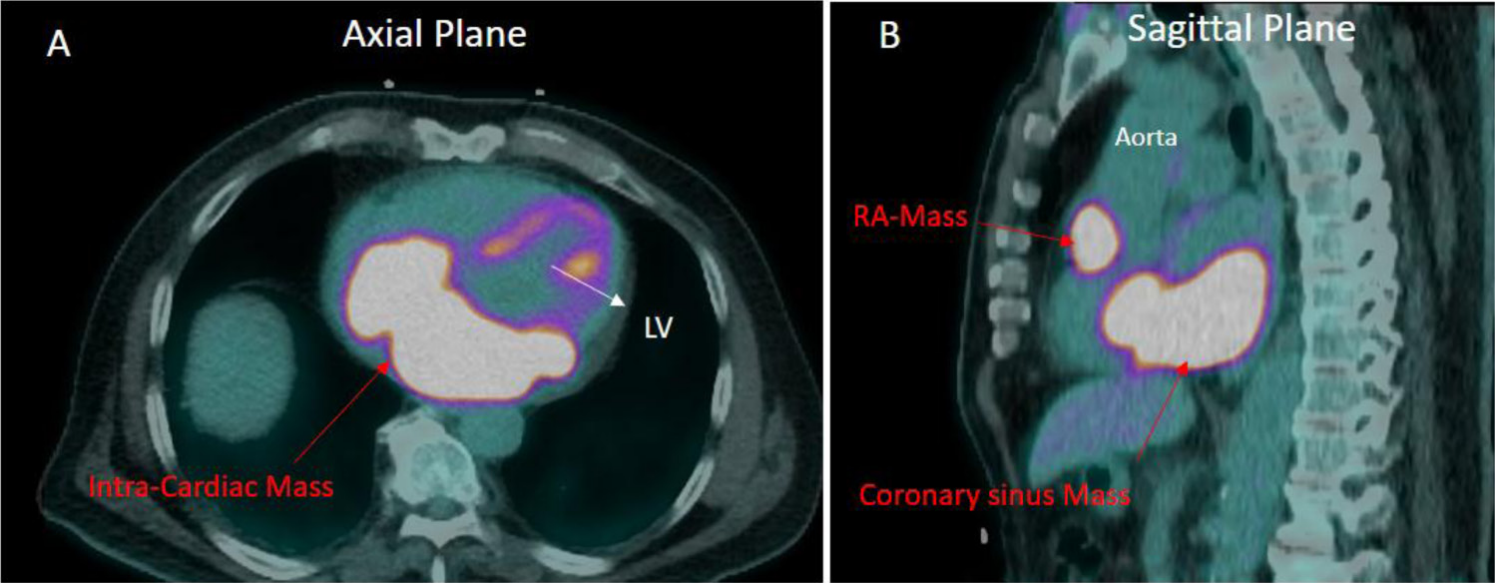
(A) Axial plane image demonstrating intense fluorodeoxyglucose (FDG) uptake in the intracardiac mass (SUV Max 18). (B) Sagittal plane image demonstrating a second intracardiac mass in the wall of the right atrium, which also has intense FDG uptake (SUV Max 12). Intense FDG uptake in both masses is consistent with a malignant tumor. LV: left ventricle; RA: right atrium
